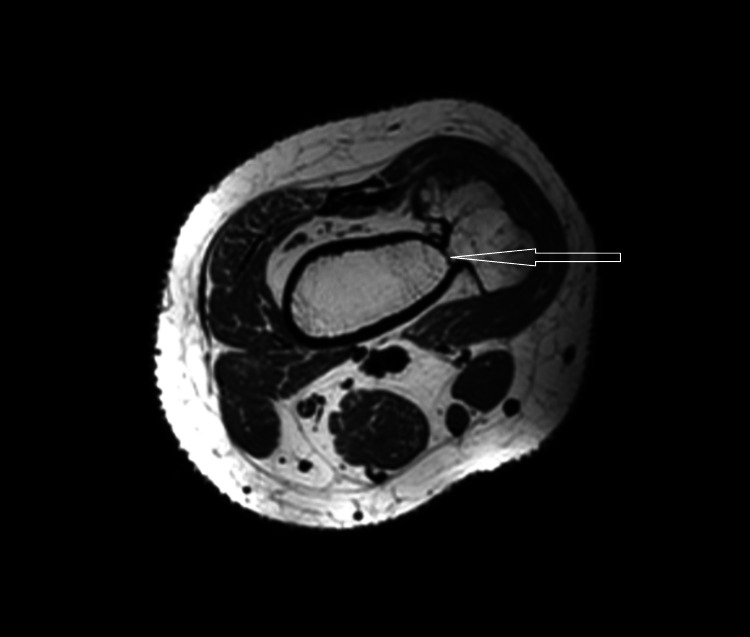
The axial T1WI image shows the continuity of the lesion with the cortex and medullary cavity of the parent femoral bone. T1W1: T1-weighted imaging
